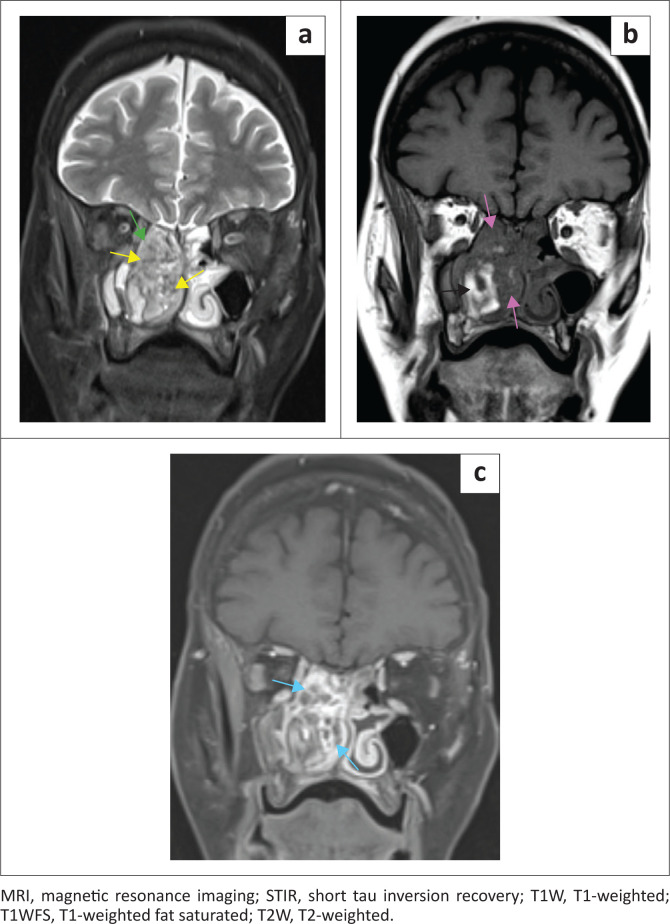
Coronal MRI STIR (a), T1W (b) and T1WFS post contrast (c). (a) Sinonasal tumour is T2W heterogeneous and hyperintense (green arrow) with T2W dark flow voids (yellow arrows). (b) T1W isointense to muscle (purple arrows) with a lateral hyperintense component (black arrow). (c) Heterogeneous post-contrast enhancement (blue arrows).
